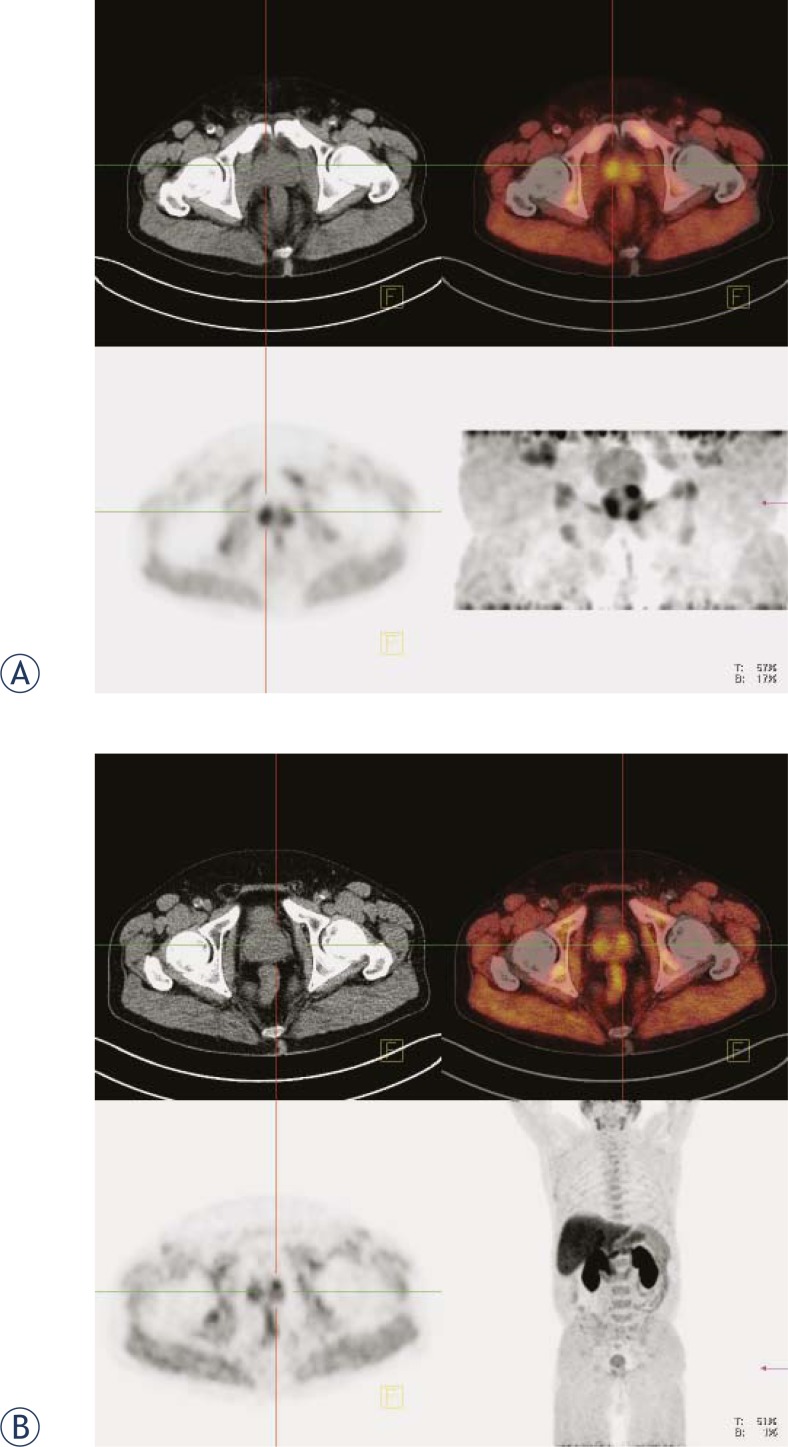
Prostate carcinoma: A prostatic bed (early images), B whole body (late images). Upper left panel: CT image. Upper right panel: fused PET/CT image. Lower left panel: PET image. Lower right panel: maximum intensity projection (MIP).
